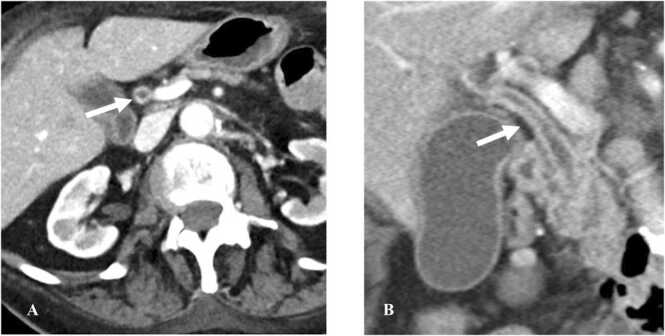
Immune-mediated cholangitis in a 72-year-old woman with melanoma on Pembrolizumab presenting with jaundice and elevated liver enzymes. A. Contrast-enhanced axial abdomen CT image shows enhancement in the wall of common bile duct (CBD) (arrow). B. Contrast-enhanced coronal abdomen CT image shows diffuse enhancement of the wall of CBD (arrow). Findings are consistent with cholangitis.
